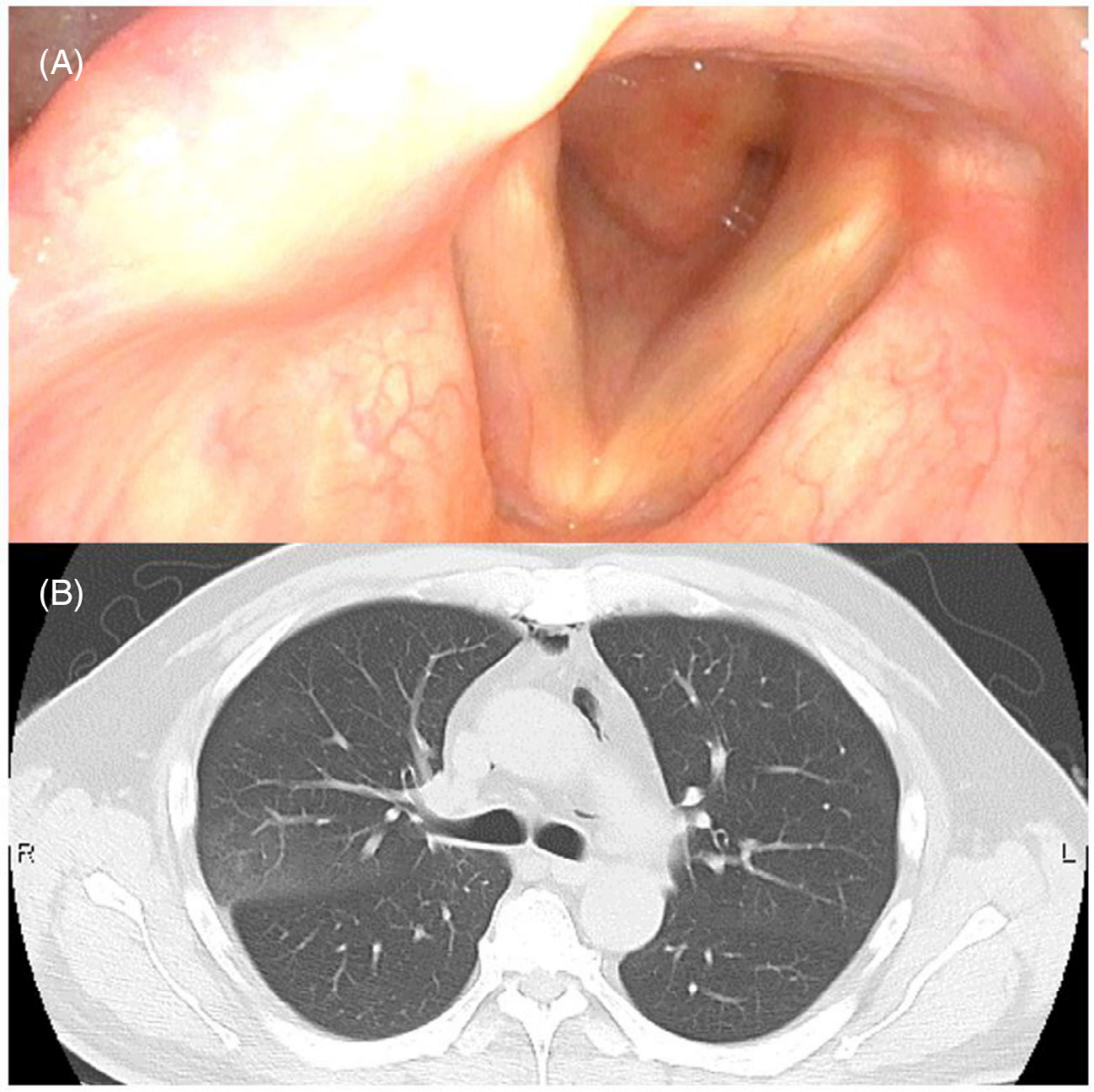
(A) Pharyngeal fibroscopy demonstrates subglottic granuloma spreading to the vocal cords. (B) An axial thoracic CT image obtained 4 days after emergent tracheostomy showing the disappearance of pulmonary edema
